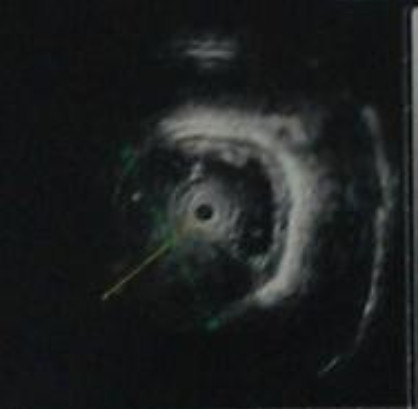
Ultrasonic gastroscopy revealed a giant mucous prominence of esophagus at 23 cm from the incisors and it originated from muscularis propria.
